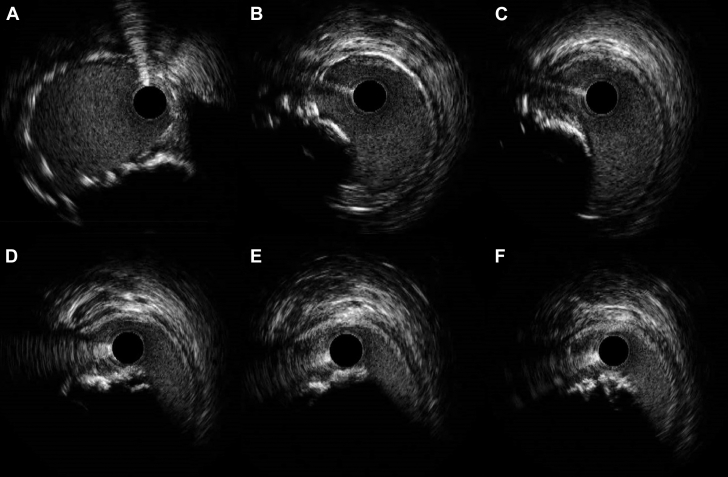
Sequential Intravascular Ultrasound Imaging of Left Main Artery (A) Intravascular ultrasound image demonstrates a patent stent in the left main artery. (B to F) Sequential intravascular ultrasound images reveal an uncovered proximal left main segment with an eccentric calcified nodule protruding into the vessel lumen and causing significant stenosis.
